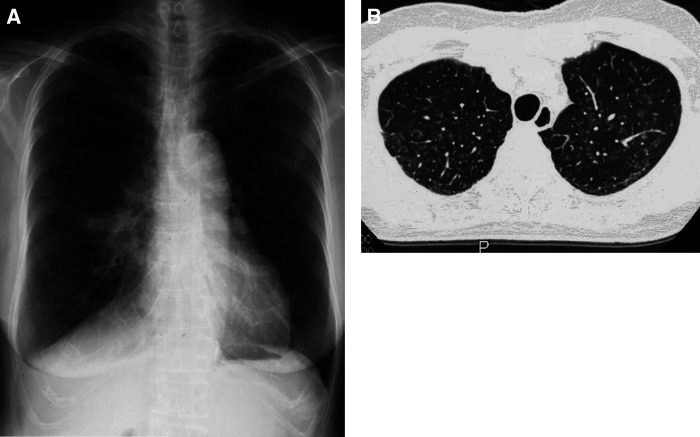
Chest X-ray (A) and CT (B) on day 30 in the patient who developed ILD. Interstitial lung disease was hardly recognisable on chest X-ray, whereas chest CT revealed a patchy, ground-glass opacity with centrilobular distribution throughout the whole lung.
